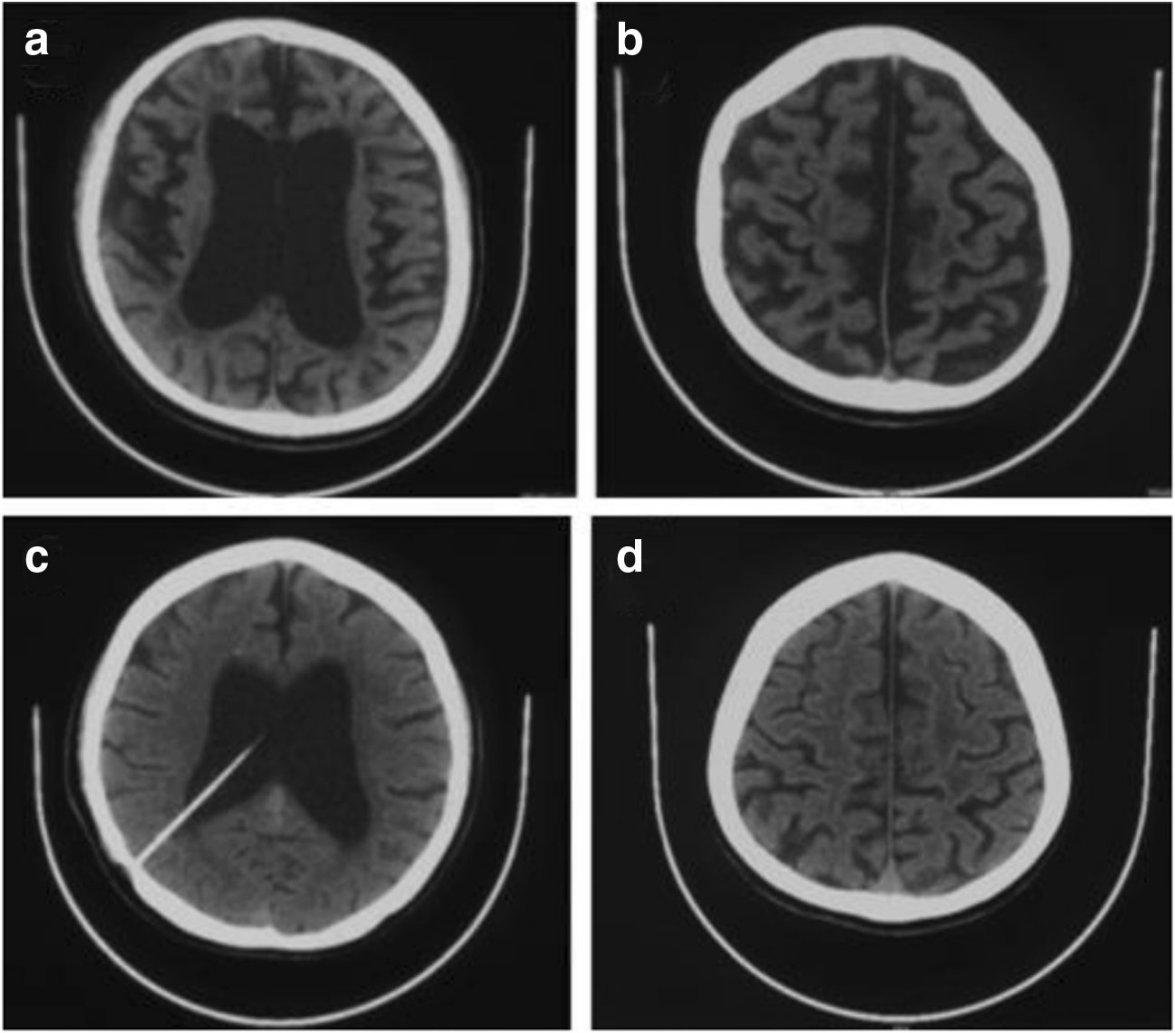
CT. CT scans show diffuse brain atrophy and enlargement of the ventricles with a small calcification located near the anterior horns of the right lateral ventricles. a, b Neuroimaging of patient III-1. c, d Neuroimaging of patient III-3
